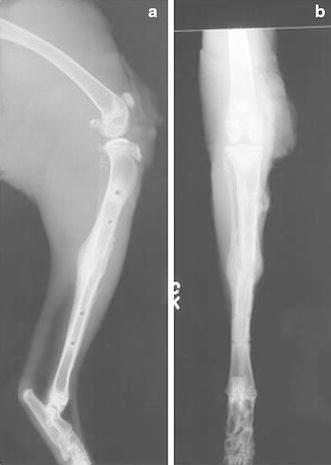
a Lateral and b AP radiographs of the tibia after removal of the fixator. Note the elliptical callus formation, the limb alignment, and the distance between the osteotomy and the pin sites
